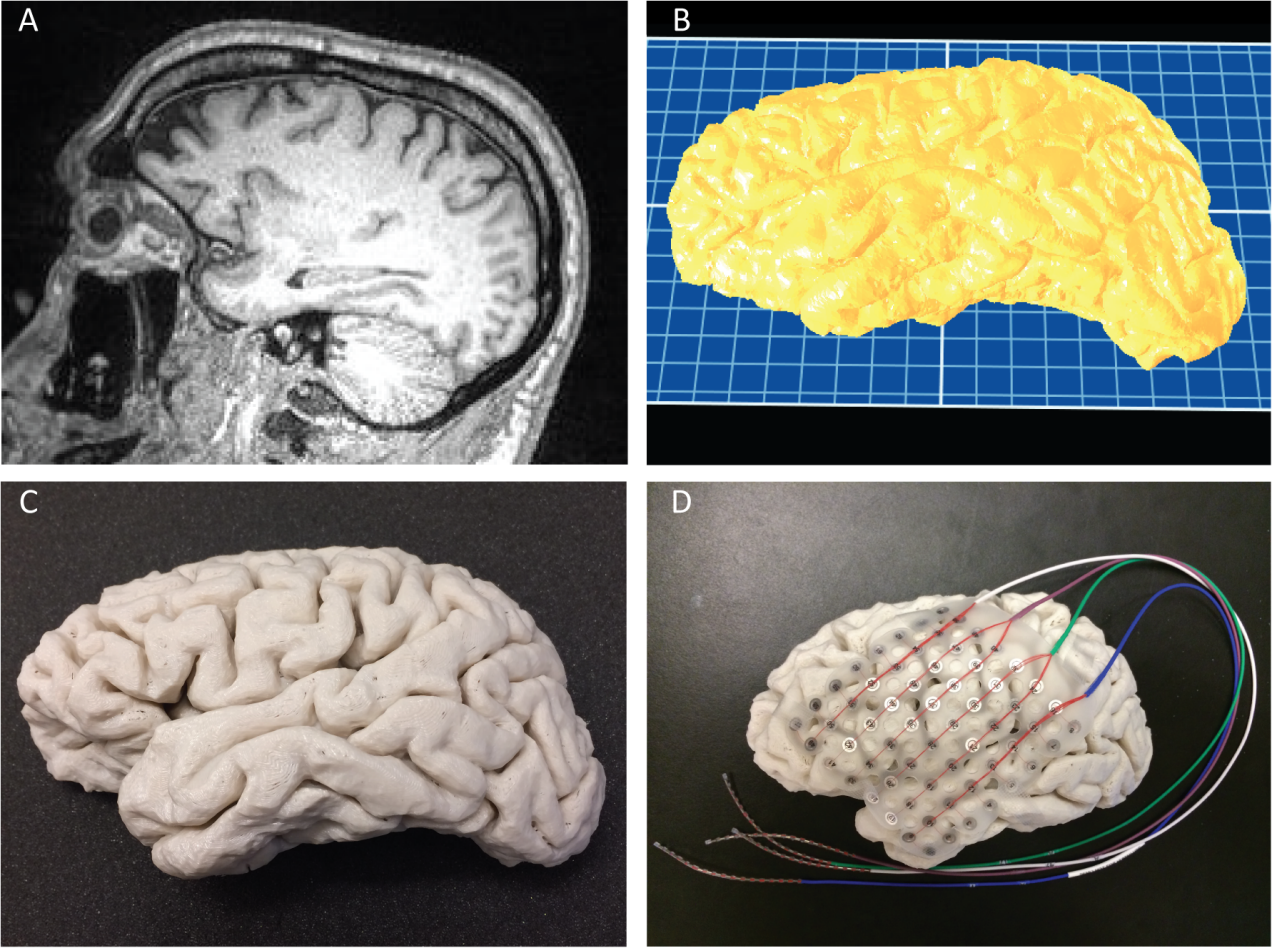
Brain Print Process. A) Sagital view of patient’s MRI (step A1). B) Brain surface rendered in ReplicatorG at the time of gcode generation (step B1). C) 3D printed brain after manually removing the support material (step B3). D) 3D printed brain overlaid with a 64-contact electrode grid to highlight possible electrode coverage during neurosurgical planning.
